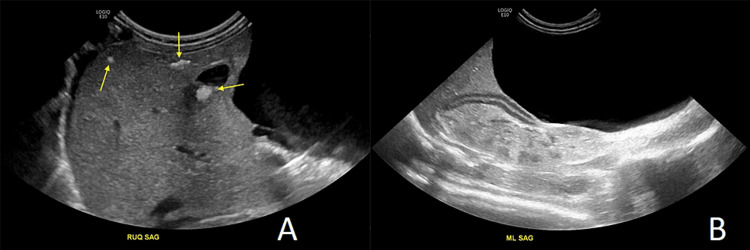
Grayscale sonographic evaluation of the abdomen. The images show multiple hyperechoic foci in the liver (arrows), suggestive of parenchymal calcifications that correspond to those seen on the abdominal x-ray (A). A large anechoic cyst occupying nearly the entire hemiabdomen was also noted (B). RUQ SAG: right upper quadrant sagittal; ML SAG: midline sagittal
